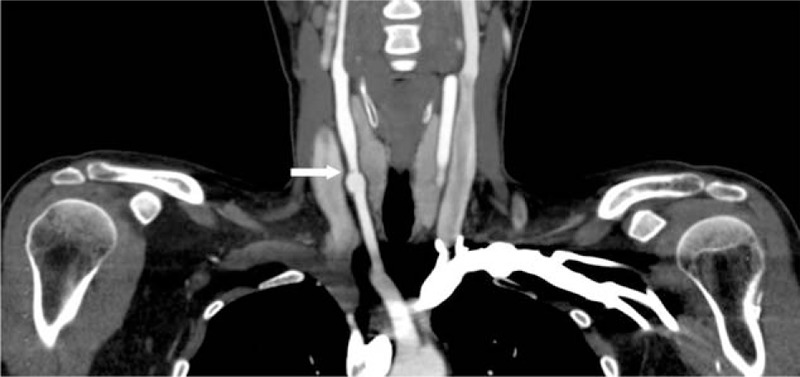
CTA showed a transversed, low density strip (arrow), causing luman stenosis, with proximal part aneurysmal enlargement on lower part of right CCA. CCA = common carotid artery, CTA = computed tomography angiography.
